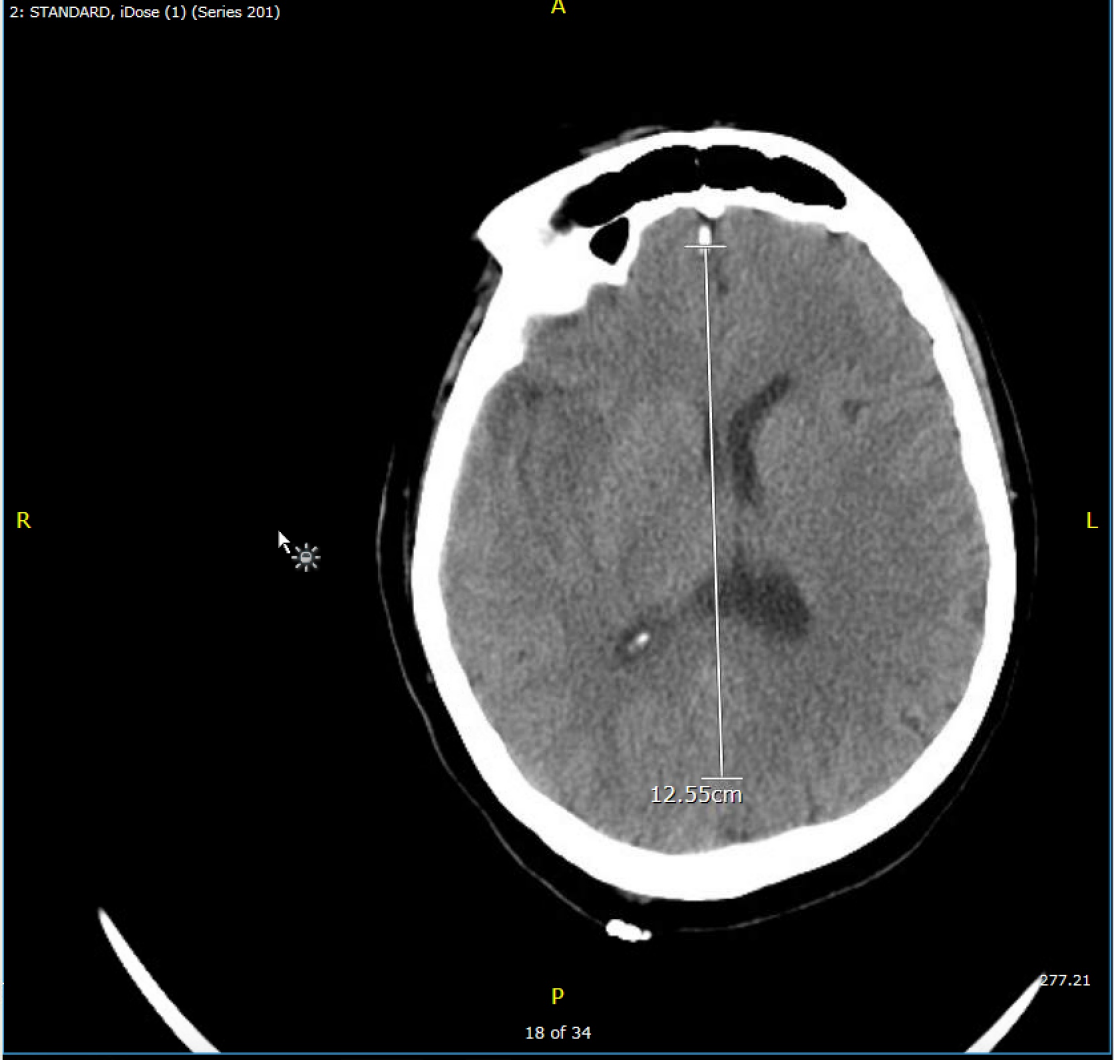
Head computed tomography (CT) scan CT of the head shows a significant acute right middle cerebral artery infarct with significant gyral swelling, mass effect, loss of gray/white differentiation in right medial temporal/insular cortex, and an external capsule with a leftward midline shift of 4 mm.
